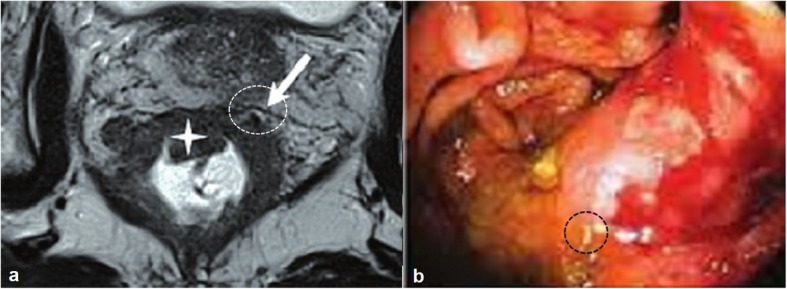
52 years old female patient with colonic extramural invasion. a: Computed tomographic colonography, the white circle indicates colonic extramural invasion. b. Colonoscopy, black circle indicates colonic extramural invasion
